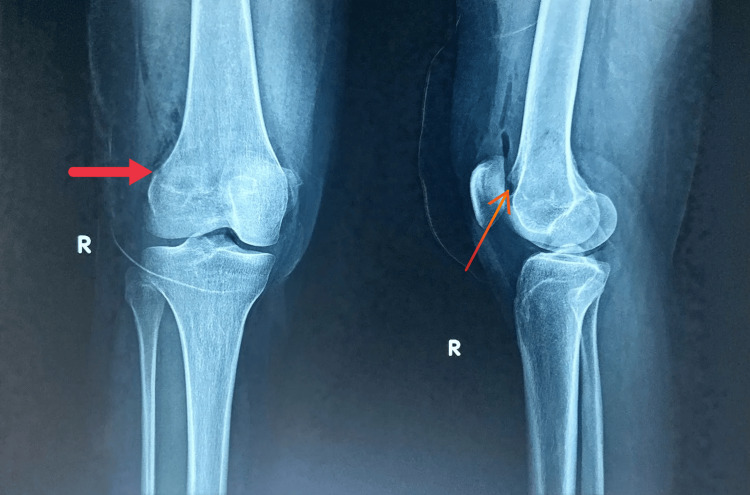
Postoperative X-ray of the right knee The red arrow shows adequate curettage of the lesion postoperatively.
